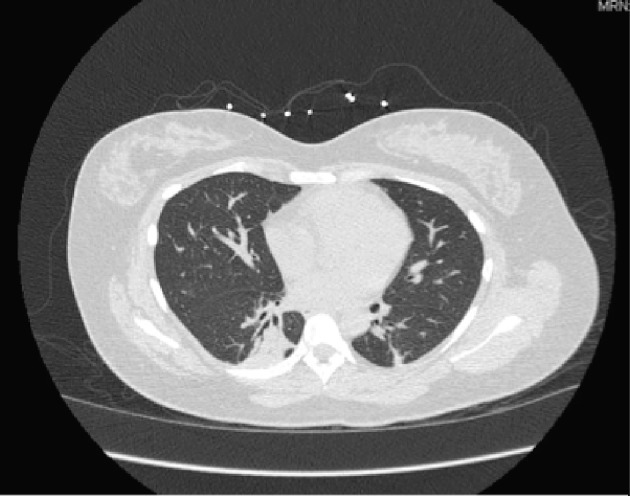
Chest CT without contrast: consolidative changes of lower lobes.
